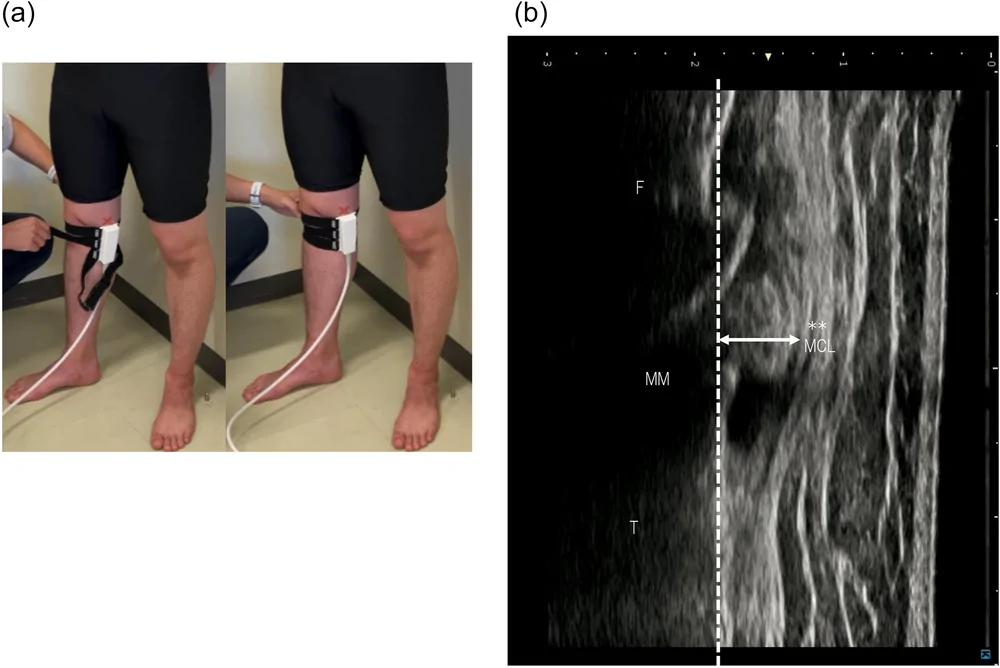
Representative ultrasound images with a prototype transducer. (a) Transducer attachment, and (b) the representative image of medial meniscus extrusion. The white line and double arrows represent the cortical line of the tibial plateau and distance of the extruded medial meniscus, respectively. F, femur; MCL, medial collateral ligament; MM, medial meniscus; T, tibia.
